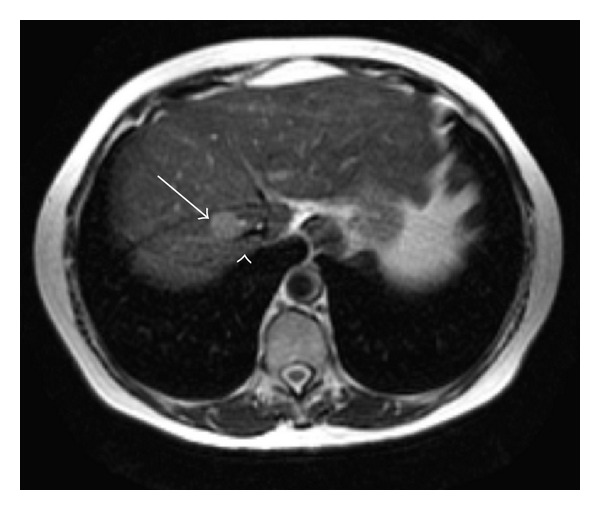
T2 weighted Magnetic Resonance image showing a tumor (arrow) compressing the RHV (arrow head).
